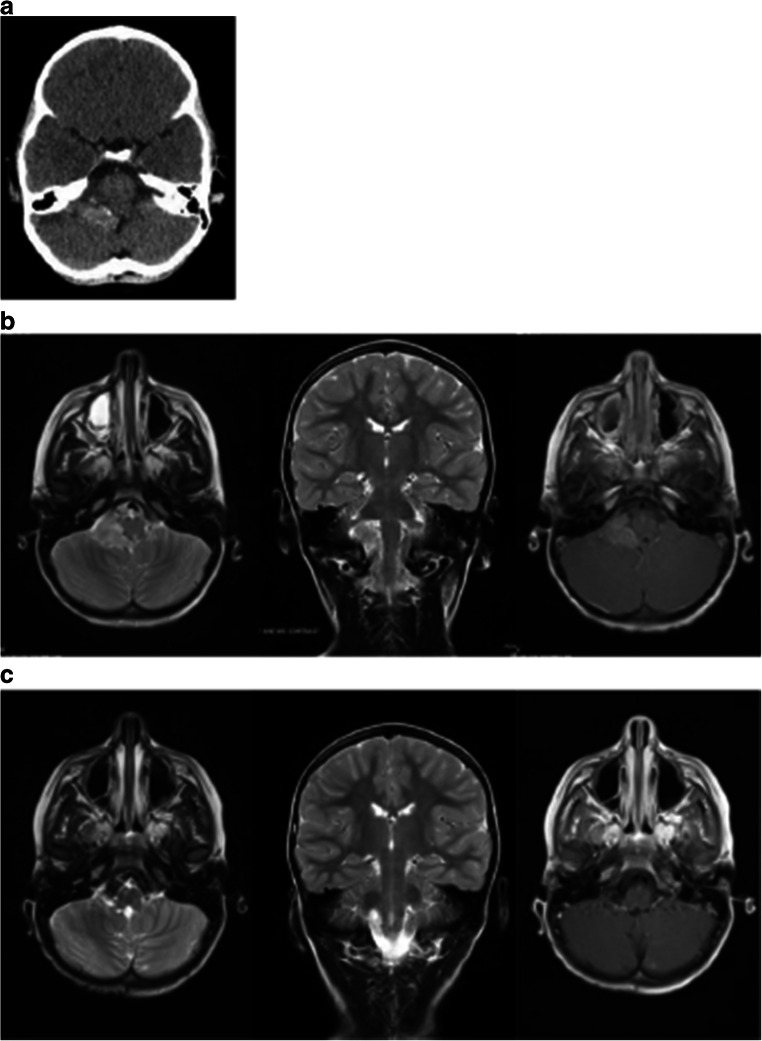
Case 8. a Non-contrast CT showing a hyperdense right cerebellopontine angle lesion centered around the foramen of Luschka. b Preoperative T2-weighted MR; axial (left) and coronal (center) images and contrast enhanced T1-weighted axial image (right) show homogeneous enhancement of a CPA choroid plexus papilloma. c Postoperative T2-weighted MR; axial (left) and coronal (center) images and T1 with contrast (right) show a resolution of a CPA choroid plexus papilloma
